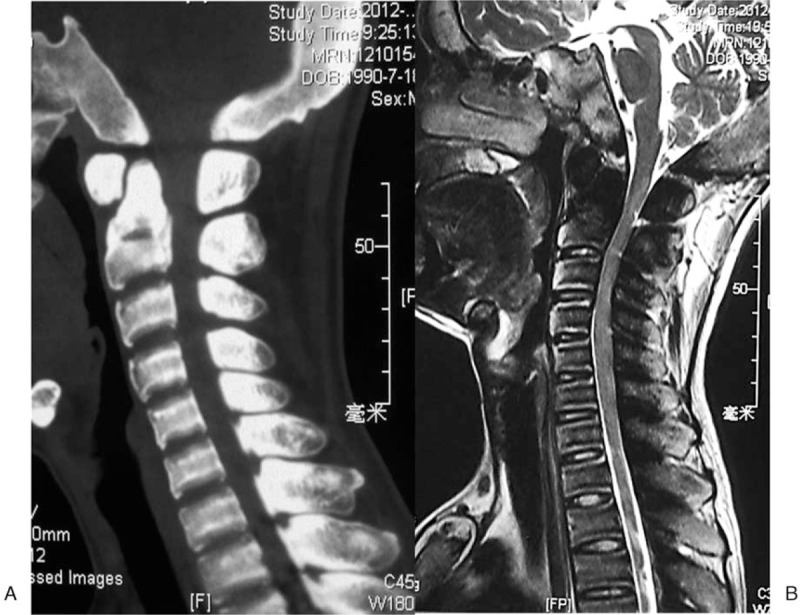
Preoperative CT and MRI of the cervical spine. (A) Preoperative CT demonstrated significant hypertrophy of bone and canal stenosis. (B) Preoperative MRI demonstrated cervical cord compression.
